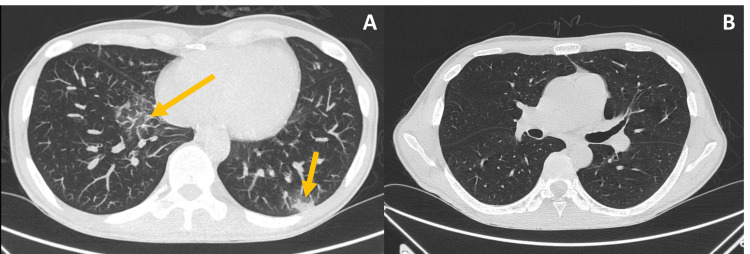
First pulmonary CT scan on day 10 after admission A - An incipient evolving inflammatory process (with arrows); B - For comparison; 1B is at the same level as the subsequent images.
